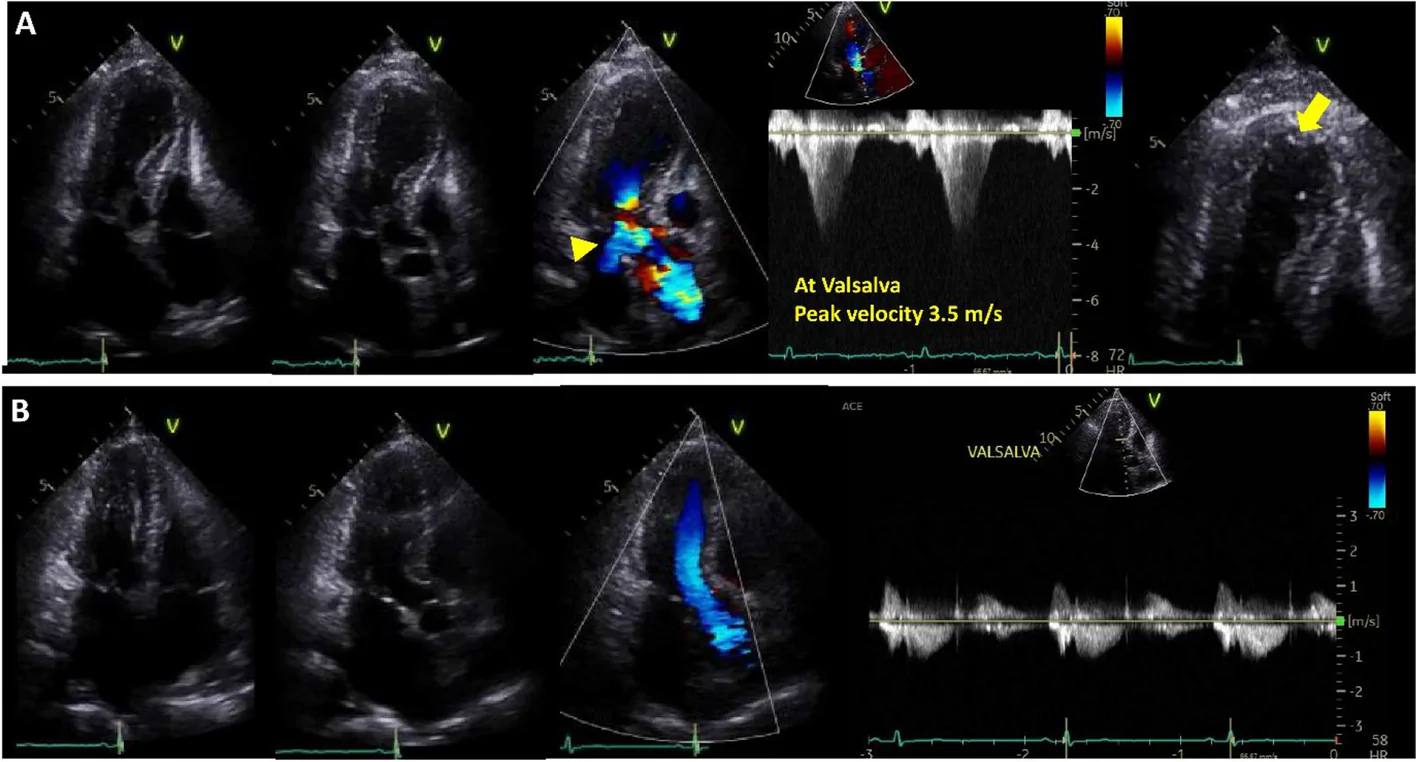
Stress induced cardiomyopathy complicated by mitral regurgitation, left ventricular outflow tract (LVOT) obstruction, and thrombus. A An 82-year-old woman was diagnosed with stress-induced cardiomyopathy (apical type). Akinetic wall motion of the apical segments of the left ventricle with hyperdynamic basal segments led to systolic anterior motion of the anterior mitral leaflet, generating mitral regurgitation (arrowhead) and dynamic LVOT obstruction. A mural thrombus was observed at the far apex (arrow). B At 4-month follow-up, wall motion abnormalities and LV systolic dysfunction had improved. No thrombus or dynamic LVOT obstruction was identified. All echocardiographic images are in end-systole
